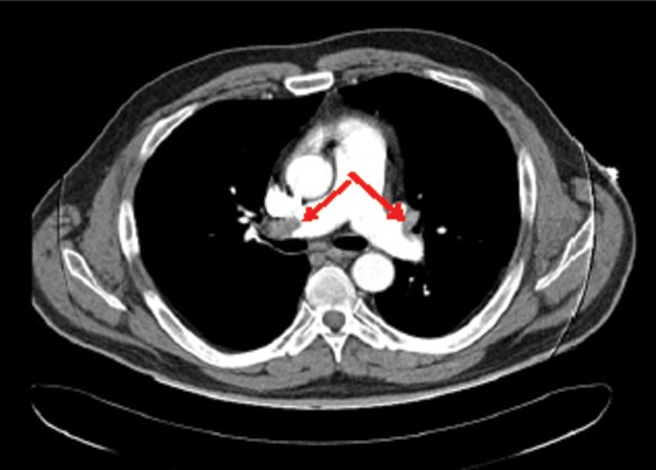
Patient 3: Axial image of CT pulmonary angiogram showing thrombi as filling defects in right main pulmonary artery (*right arrow*) extending into its branch and in distal left pulmonary artery (*left arrow*) with extension into its superior branch.
